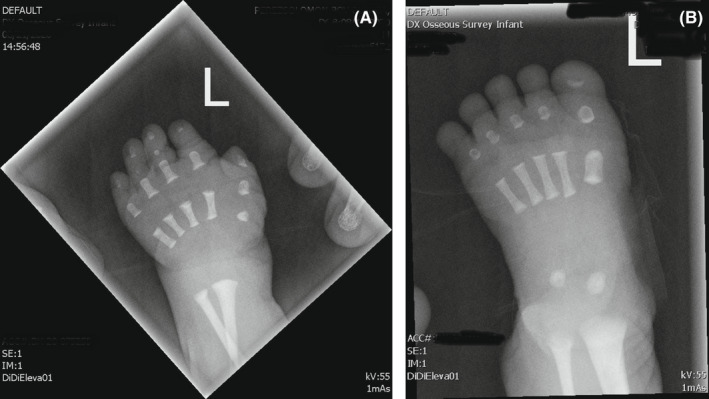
(A) Radiograph of the hand shows absence/short middle phalanges of fingers. (B) Radiograph of the foot shows absence/short middle phalanges of toes
